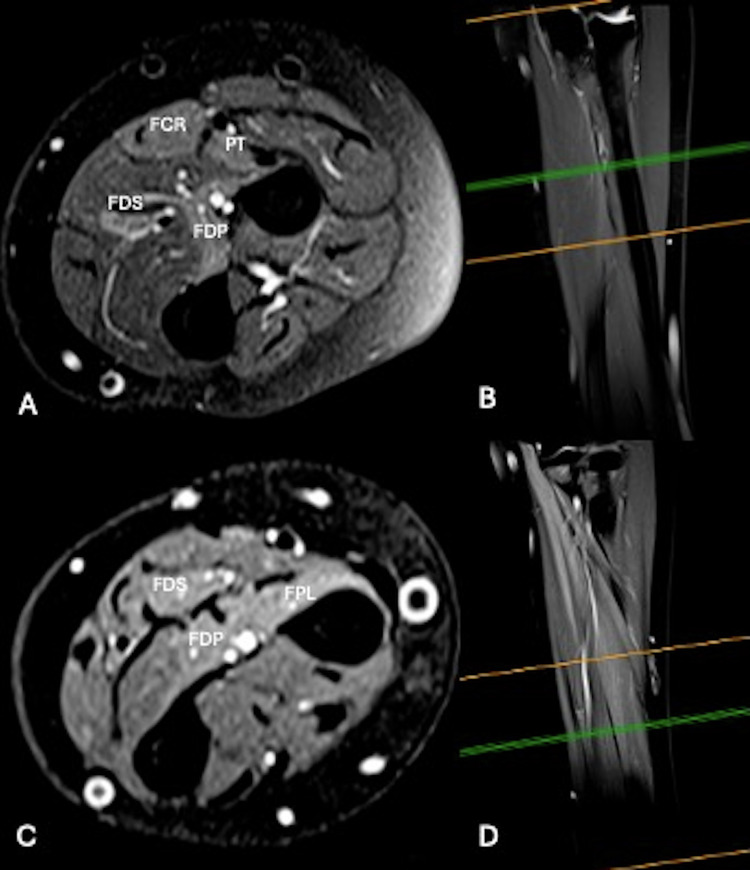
Pronator teres syndrome. Axial fat-suppressed PD MRI images (A, C) at the proximal (reference in B) and distal (reference in D) third of the forearm show edema of pronator teres (PT), flexor carpi radialis (FCR), flexor digitorum superficialis (FDS), radial half of the flexor digitorum profundus (FDP) and flexor pollicis longus (FPL) muscles due to denervation
